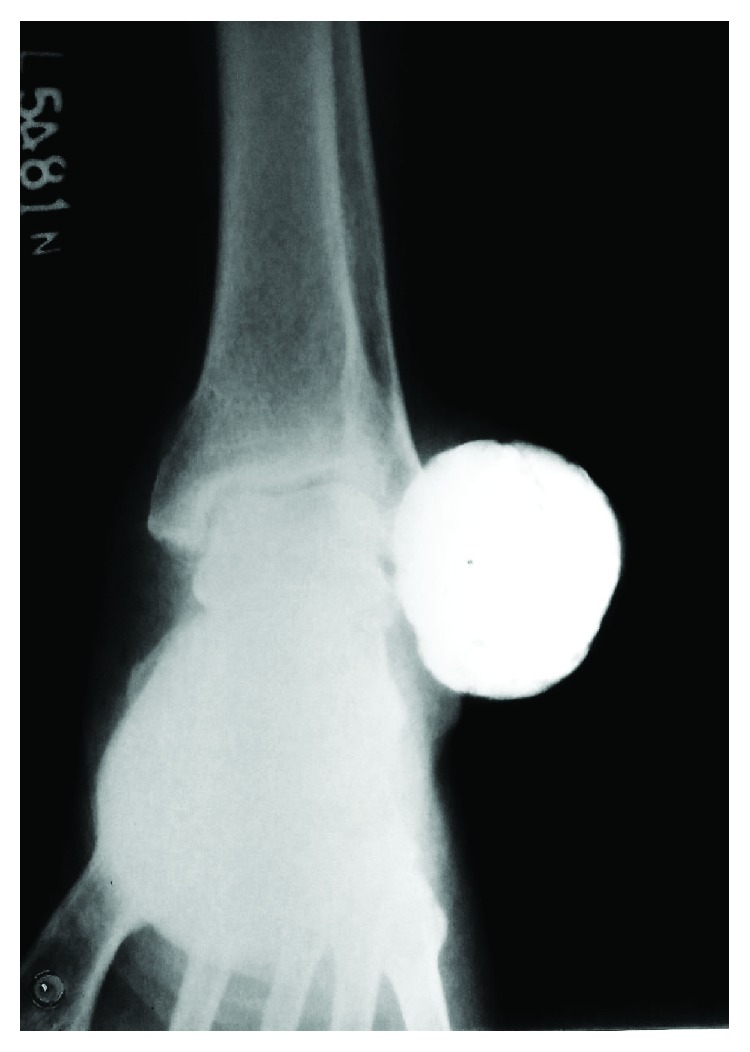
Anteroposterior X-ray of the left ankle joint showing a radiopaque mass with dense homogenous calcification over the lateral aspect of the ankle joint.
